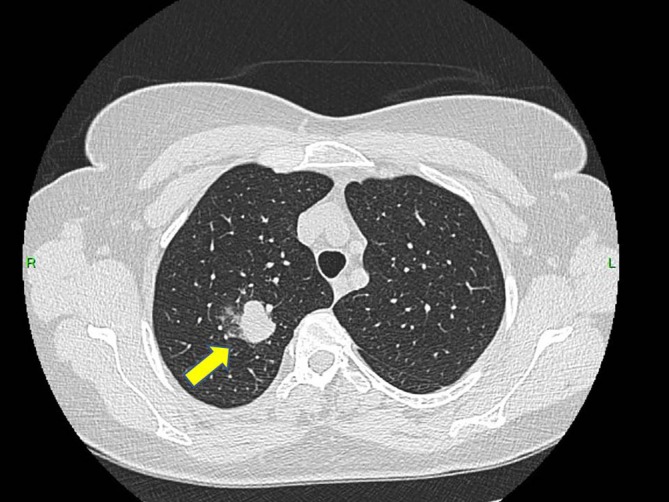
Chest computed tomography (CT) scan showing a solitary pulmonary nodule in the right upper lobe (yellow arrow), which was one of the first radiological findings raising suspicion of a thoracic malignant process.
